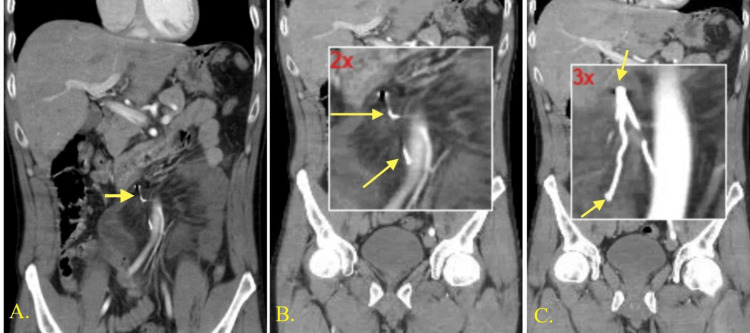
Coronal views of CT images of the abdomen with IV contrast Images 2A-2C show an IVC filter wire strut measuring 25 mm penetrating the inferior vena cava into the peritoneal cavity. Image 2A shows bowel wall changes surrounding the swirl of mesentery and small bowel volvulus. Image 2B shows a piece of IVC filter wire broken off and in the mesentery of the small bowel. Image 2C shows magnification of the wire strut. CT, computed tomography; IV, intravenous; IVC, inferior vena cava
